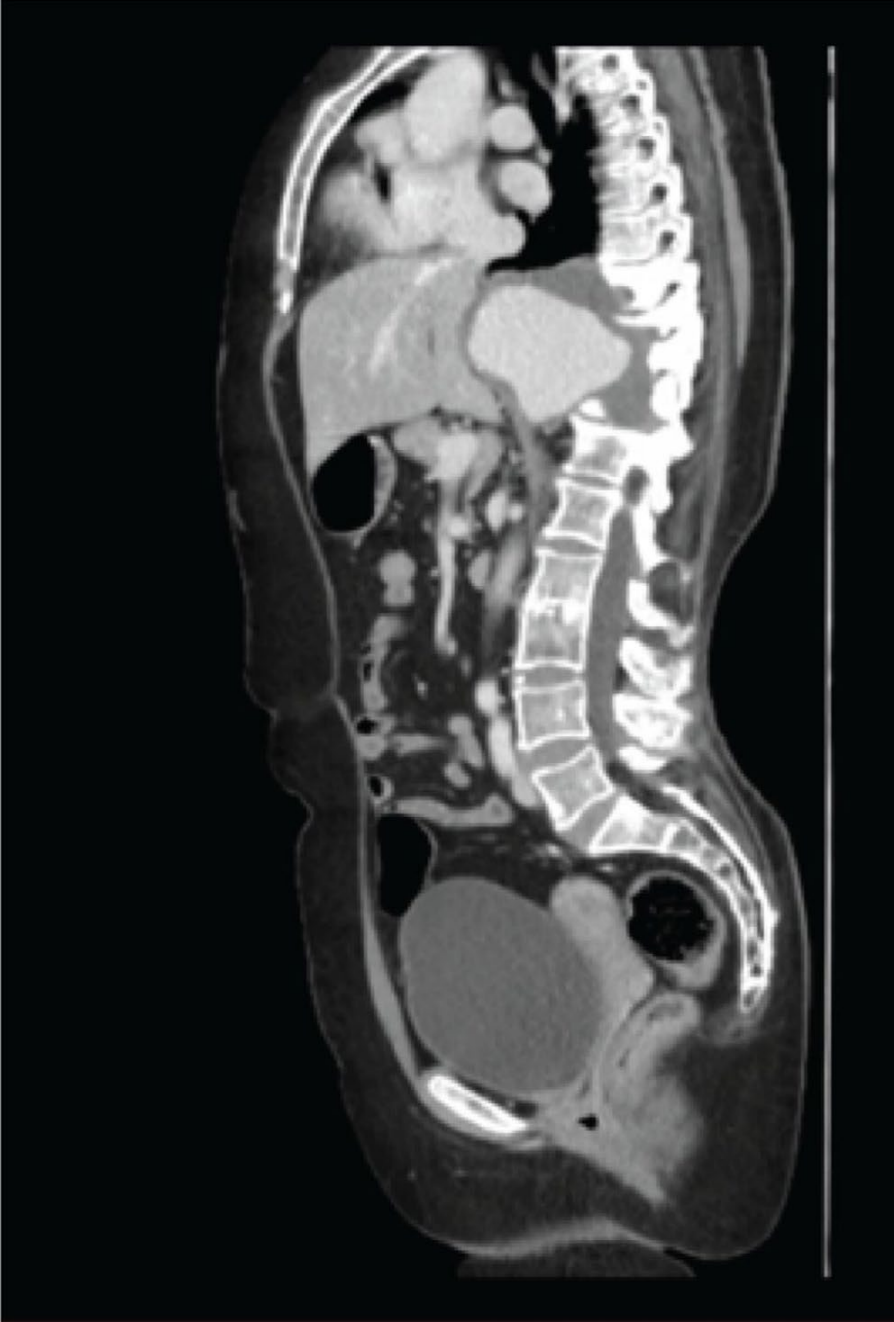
3D CT reconstruction of the thoracolumbar spine and aorta showing severe kyphotic deformity due to vertebral collapse. The spatial relationship between the aneurysm and vertebral elements is clearly visualized.
